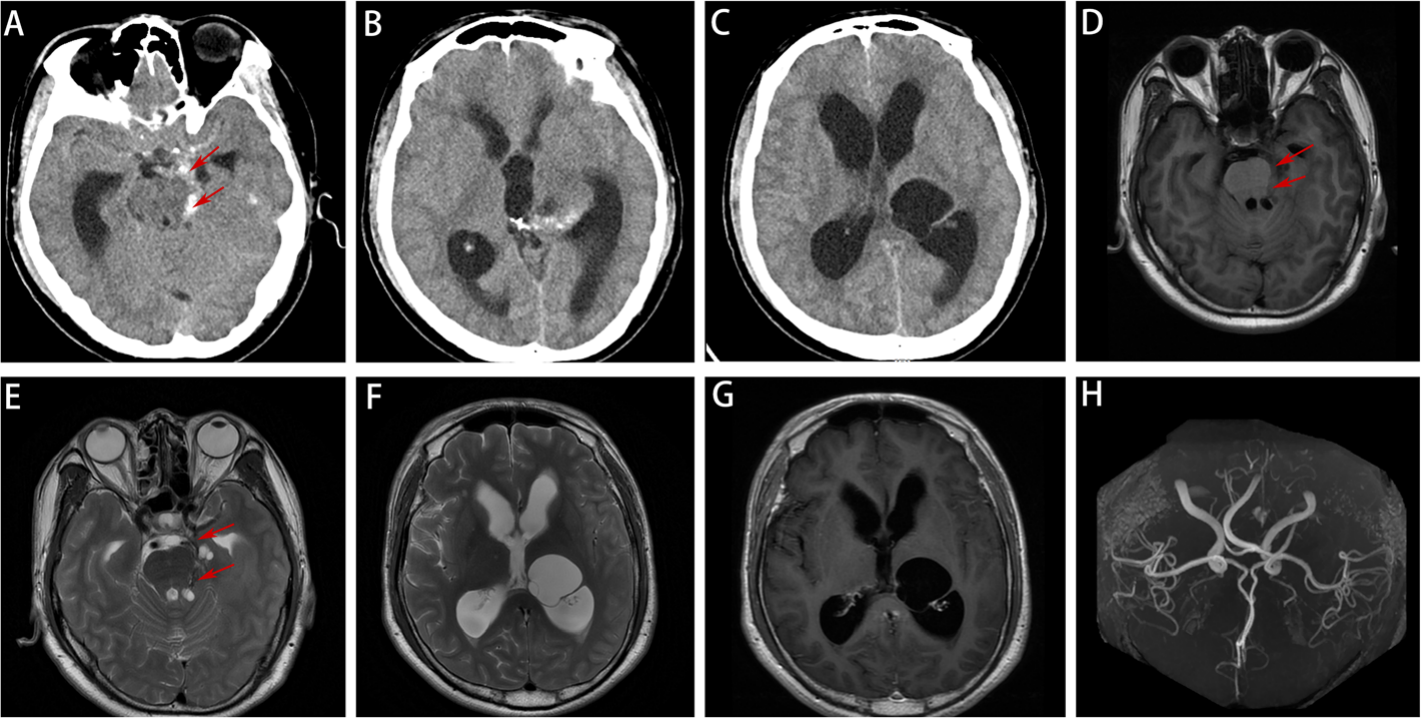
CT scan images showed high-density shadows in cisterna circinata cerebri were seen (A), and bilateral lateral ventricle and third ventricle dilatation (B). Multiple cystic lesions were seen in the third ventricle, the left basal ganglia region, the left hippocampal region (C). MRI results showed that T1 and T2 presented low signal (D, E). T2 presented high signal in the third ventricle, the left basal ganglia region, the left hippocampal region (F), with no significant enhancement after contrast-enhanced scanning (G). Furthermore, MRA indicated no aneurysms found in intracranial arterial system (H)
